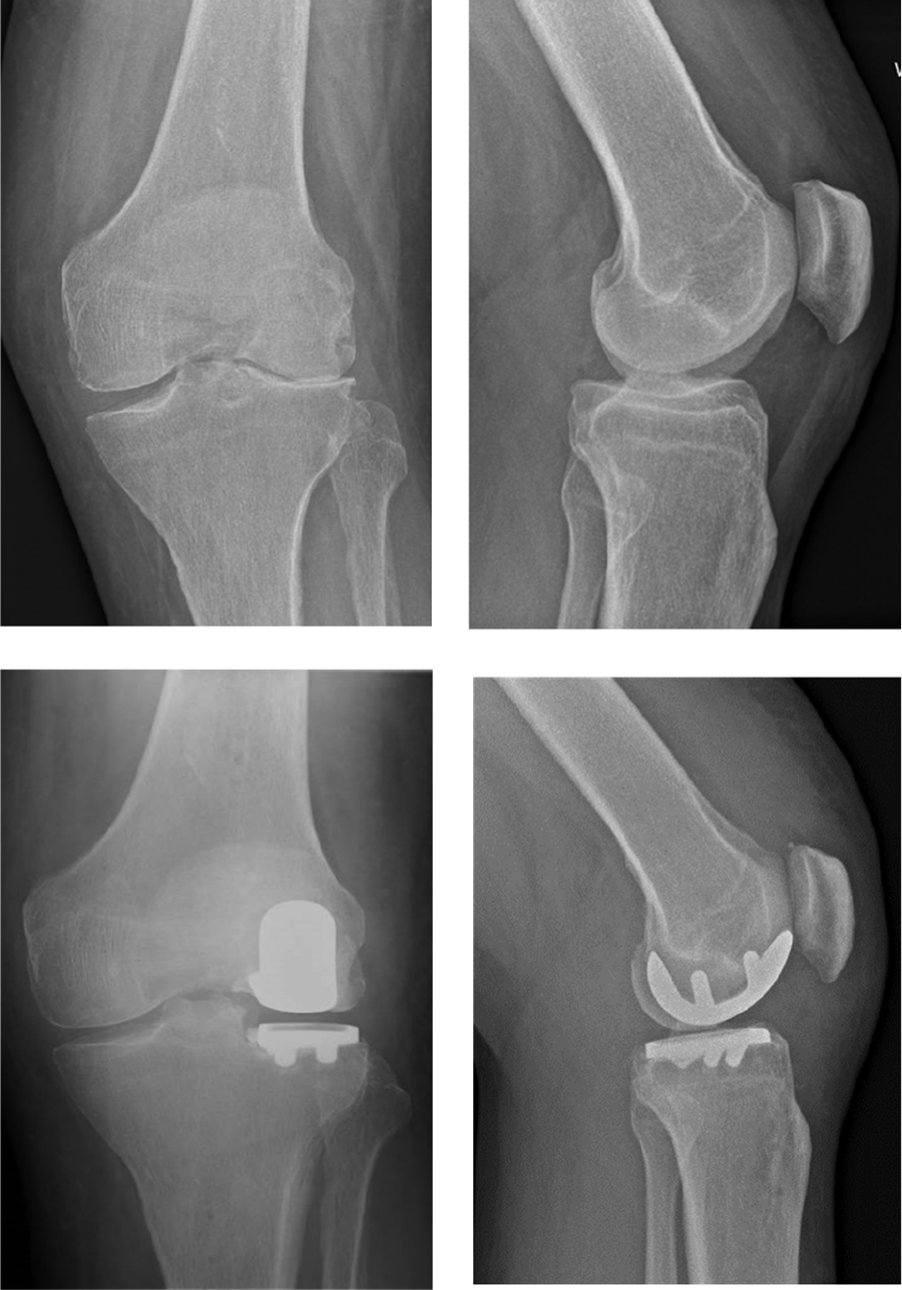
Pre- and post-operative radiographs depicting lateral UKA prosthesis.
